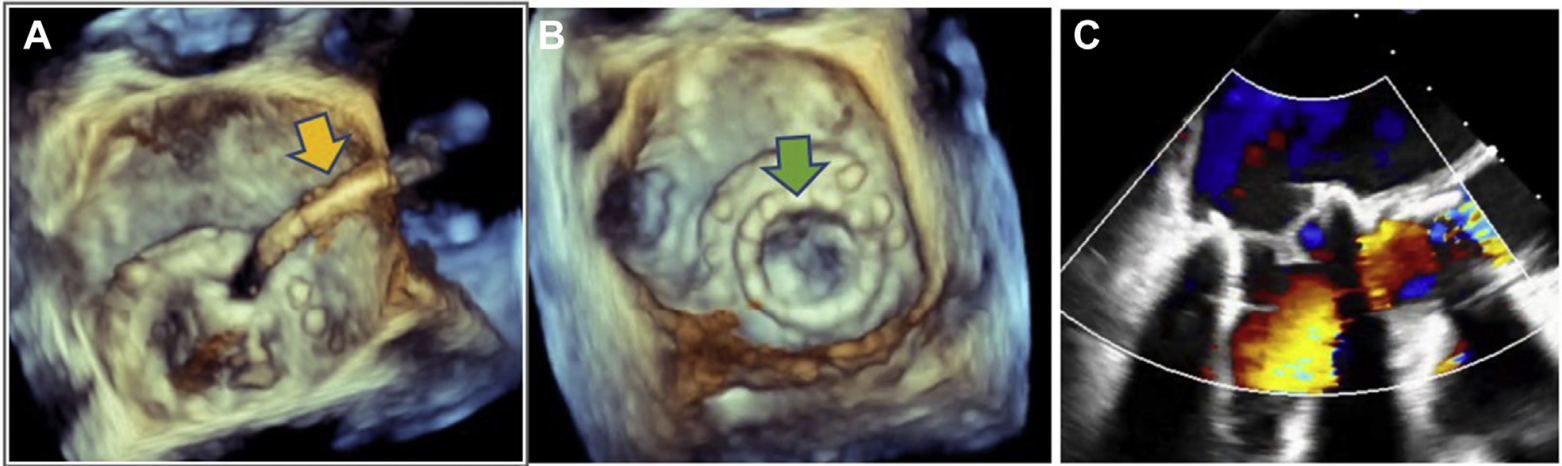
Transesophageal echocardiogram showing the new transcatheter mitral valve-in-valve transseptal delivery system (A, *yellow arrow*) and replacement transcatheter heart valve (B, *green arrow*). (C) New competent mitral valve without regurgitation.
